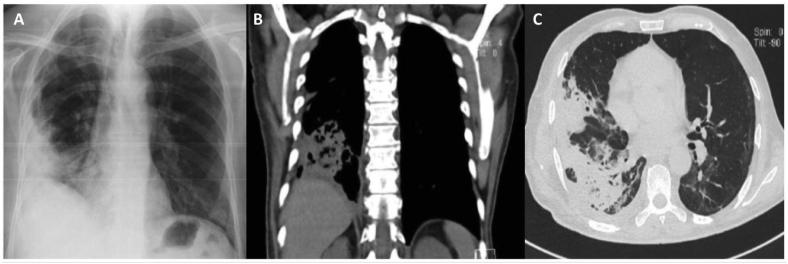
A) Posteroanterior chest X-ray: right basal heterogeneous opacity. B) Chest CT: hyperdense image, compatible with air consolidation. C) Chest CT: pulmonary opacity compatible with necrotizing pneumonia, in close contact with the pleura, with central cavitation.
